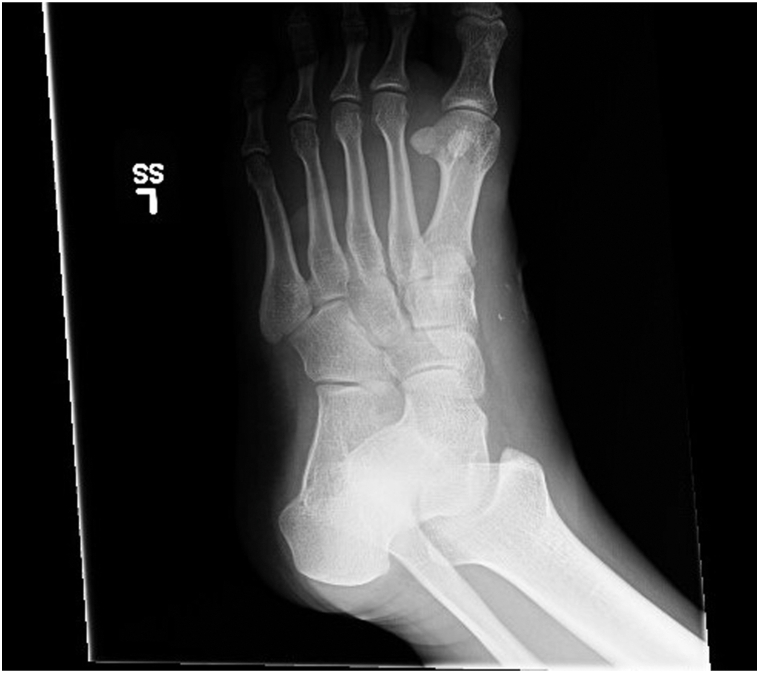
Left foot radiograph demonstrated focal radiopaque particulate material at mid-dorsal foot.
